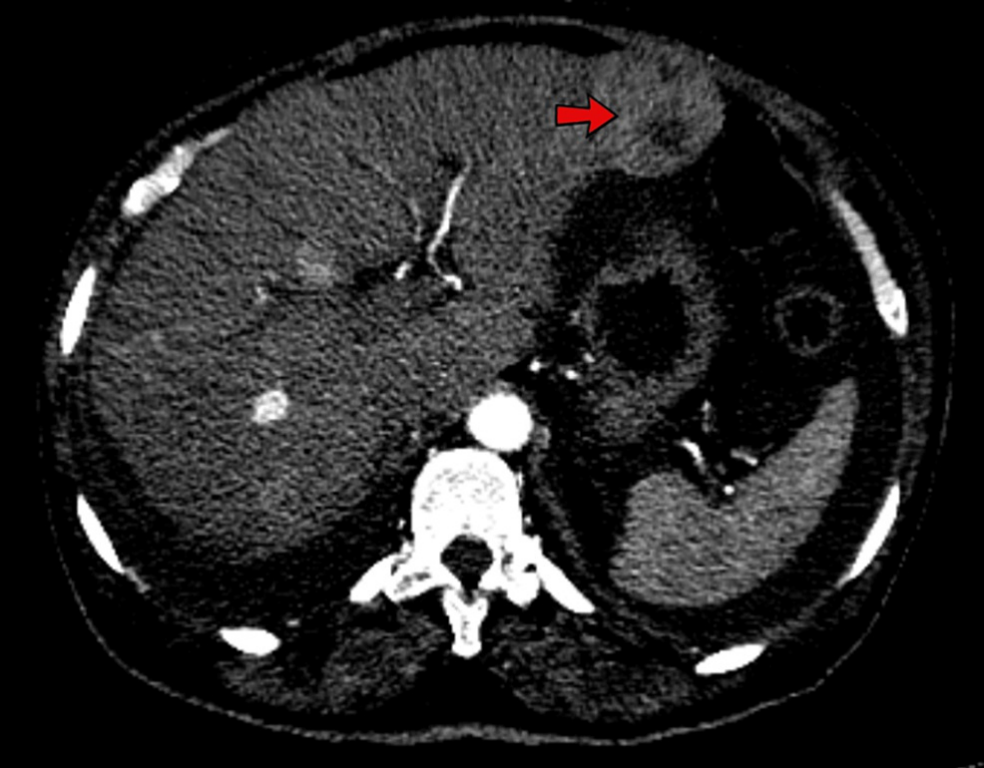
A CT scan of the chest, abdomen, and pelvis showed 4.4 x 5 cm of liver mass in the left lobe.
